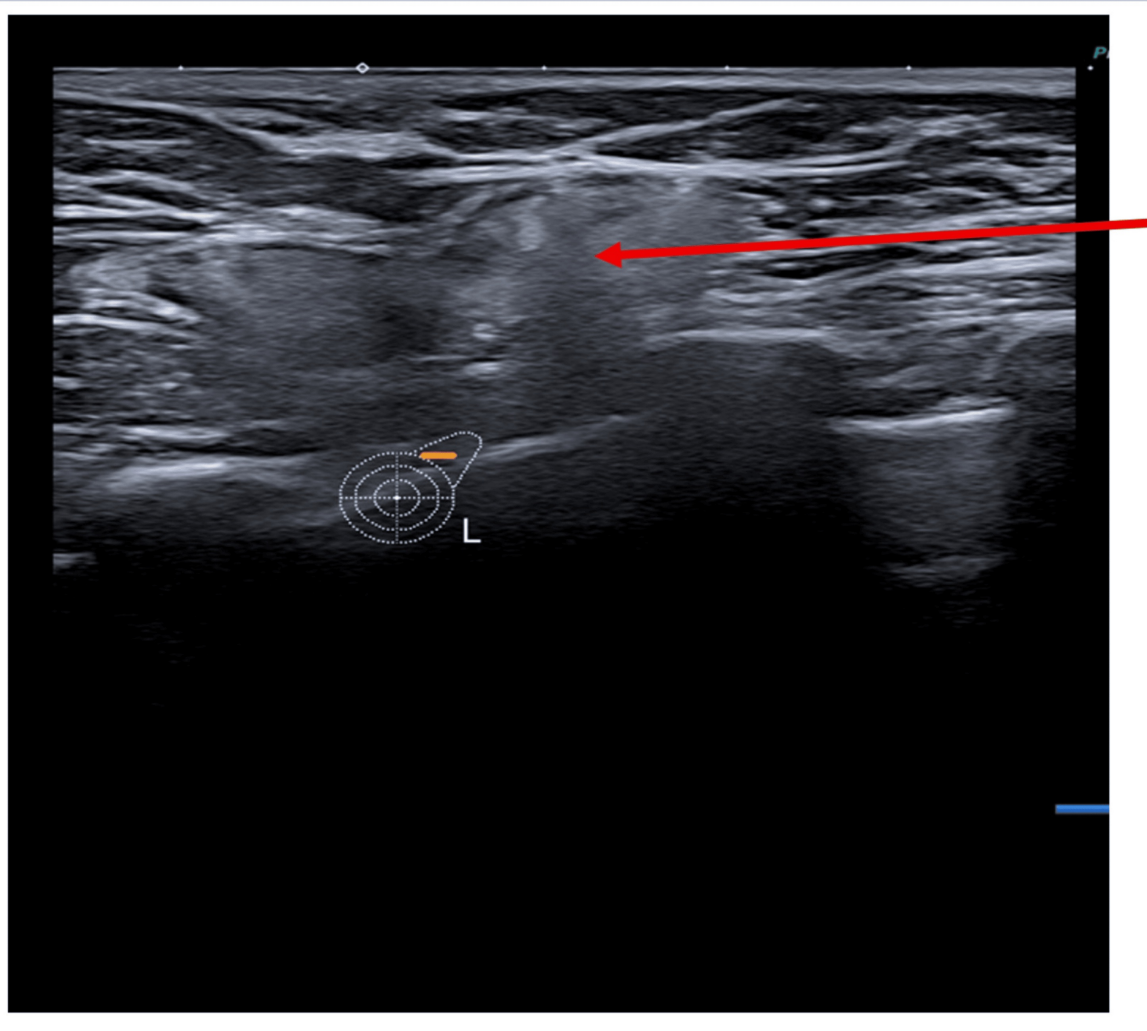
The ultrasound scan of the left axilla revealed a snowstorm appearance (arrow) since lymph nodes were loaded with silicone.
